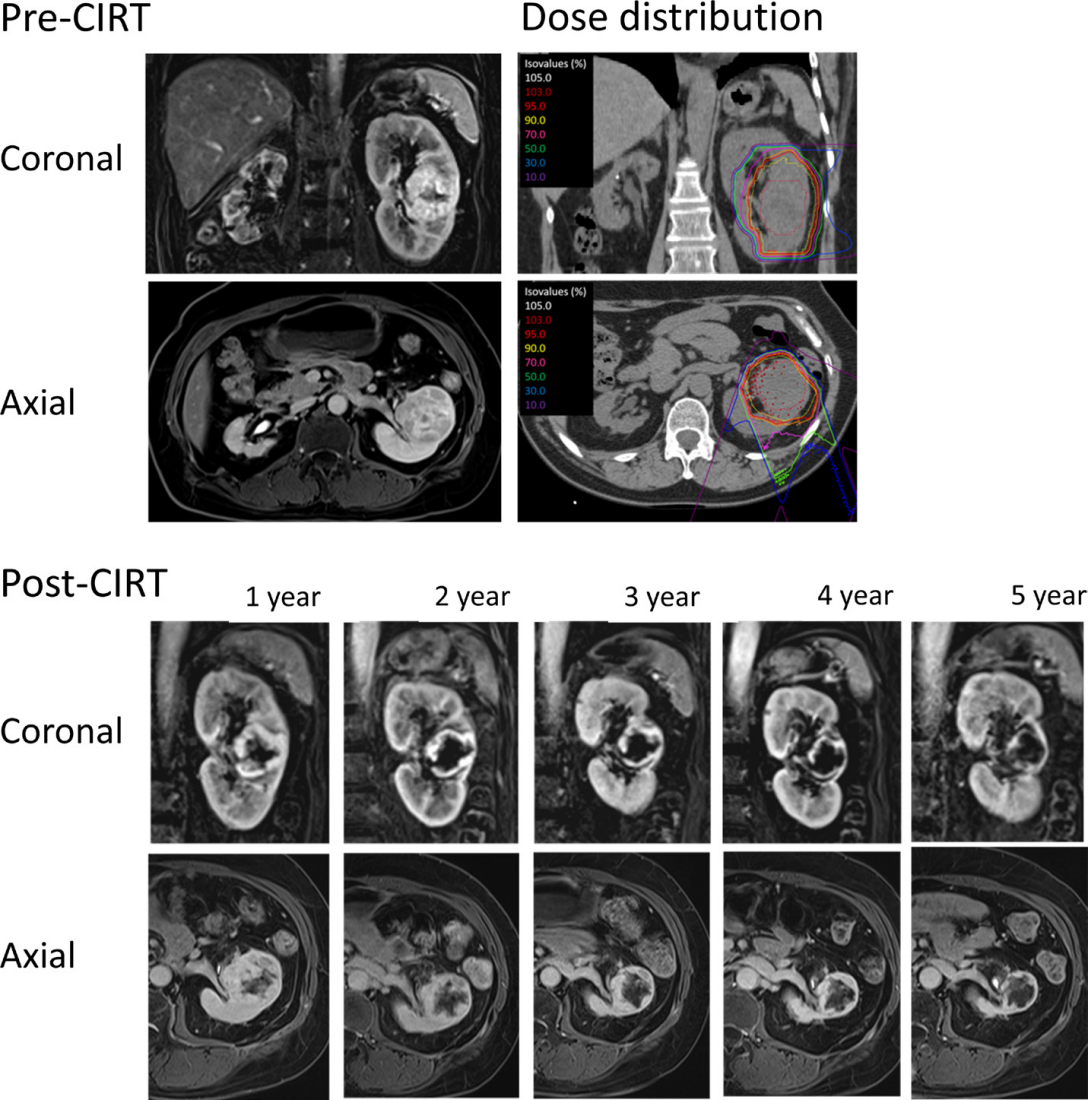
Morphologic changes of the tumor in the left kidney on contrast-enhanced axial and coronal MRI images in patient #1 at pre-, and post-CIRT at 1 to 5 years, as well as dose distribution
